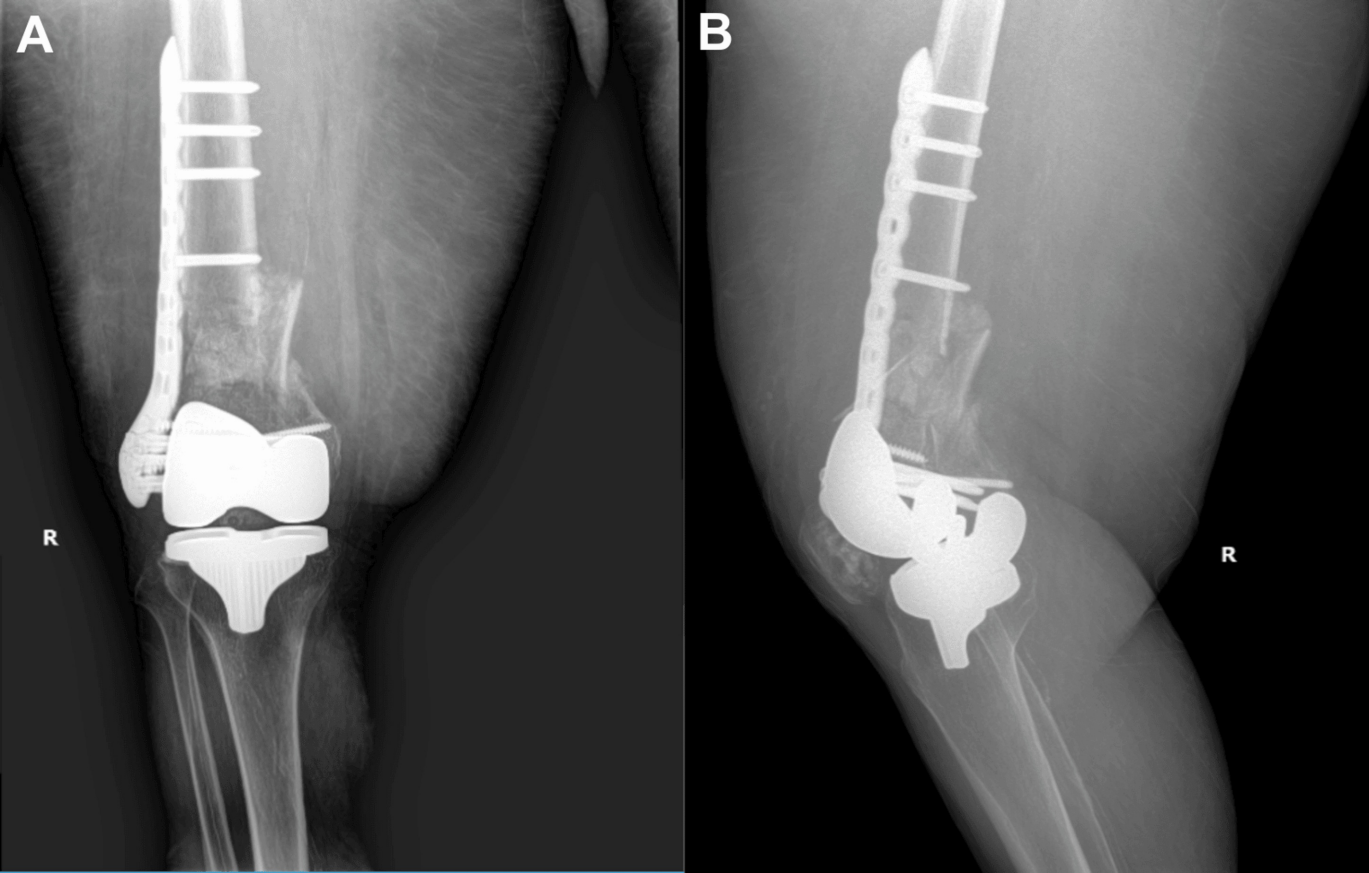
Radiographs obtained at four months postoperative following fixation of a periprosthetic distal femur fracture (A) Anteroposterior and (B) oblique radiographs of the right femur taken four months after open reduction and internal fixation of a comminuted periprosthetic distal femoral fracture proximal to a TKA implant. A lateral distal femoral locking plate was used for fixation. The TKA components remained well-aligned with no signs of loosening, and callus formation is evident.
